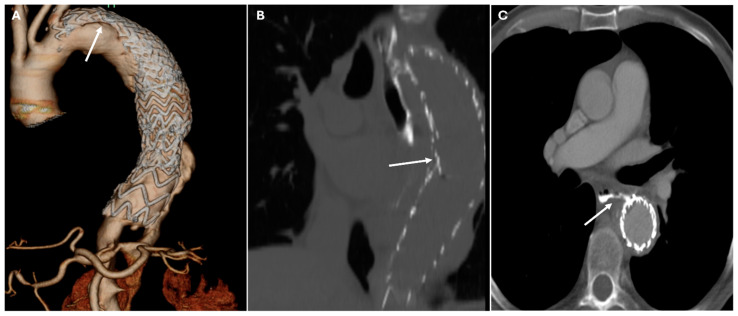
CT images of complications of TEVAR. Panel (A) shows the caudal migration of the stent graft in the thoracic descending aorta with an endoleak type IA (white arrow). Panel (B) depicts a type III endoleak (white arrow). The endoleak, which occurs at the junction of the stent graft and native aorta, leads to persistent blood flow into the false lumen, causing significant complications such as the aorta-esophageal fistula seen in Panel (C) (white arrow). This condition requires urgent intervention due to the risk of hemorrhage and gastrointestinal complications.
